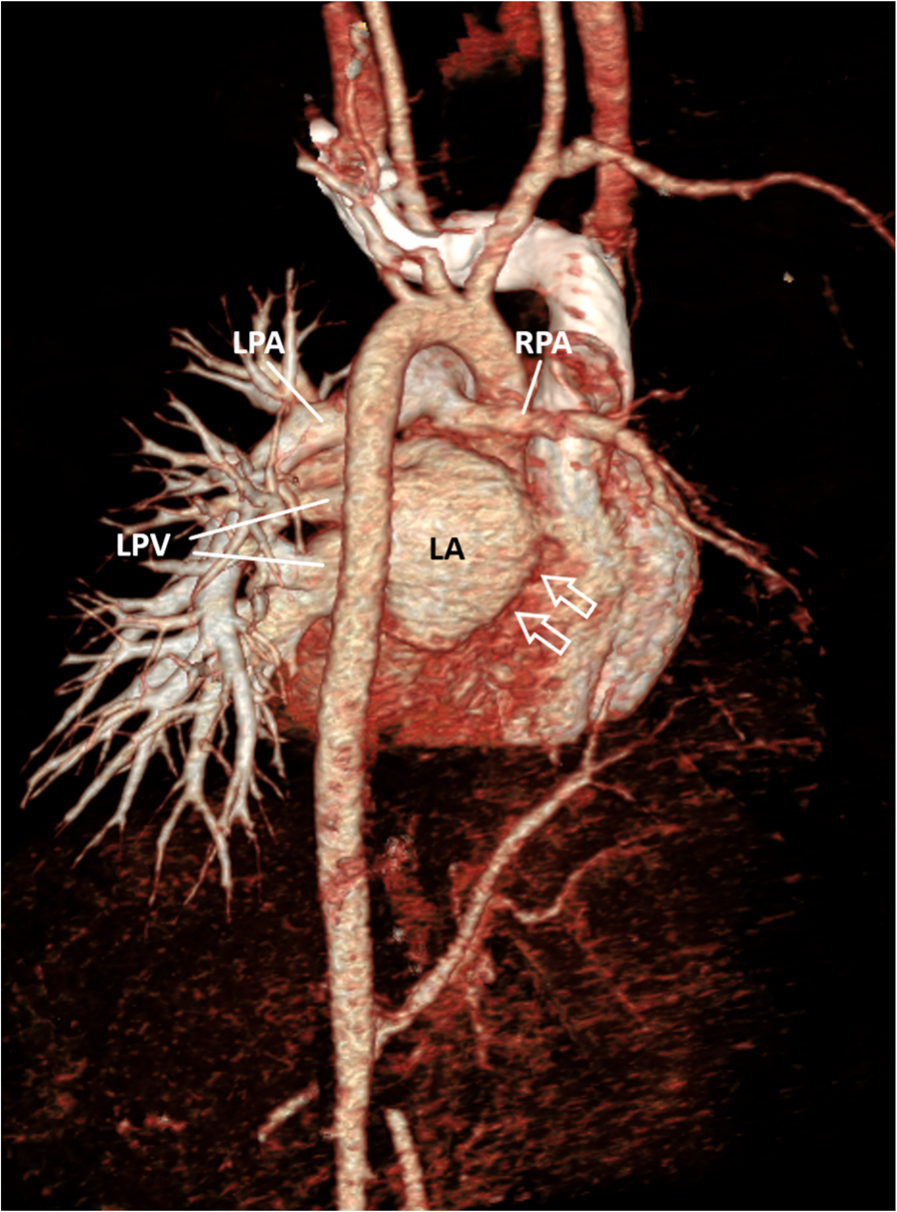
Volume-rendered reconstructed image of cardiac CT showing absence of right pulmonary veins with smooth appearance of adjacent posterior left atrial wall (white arrows). LA = left atrium, RPA = right pulmonary artery, LPA = left pulmonary artery, LPV = left pulmonary veins
